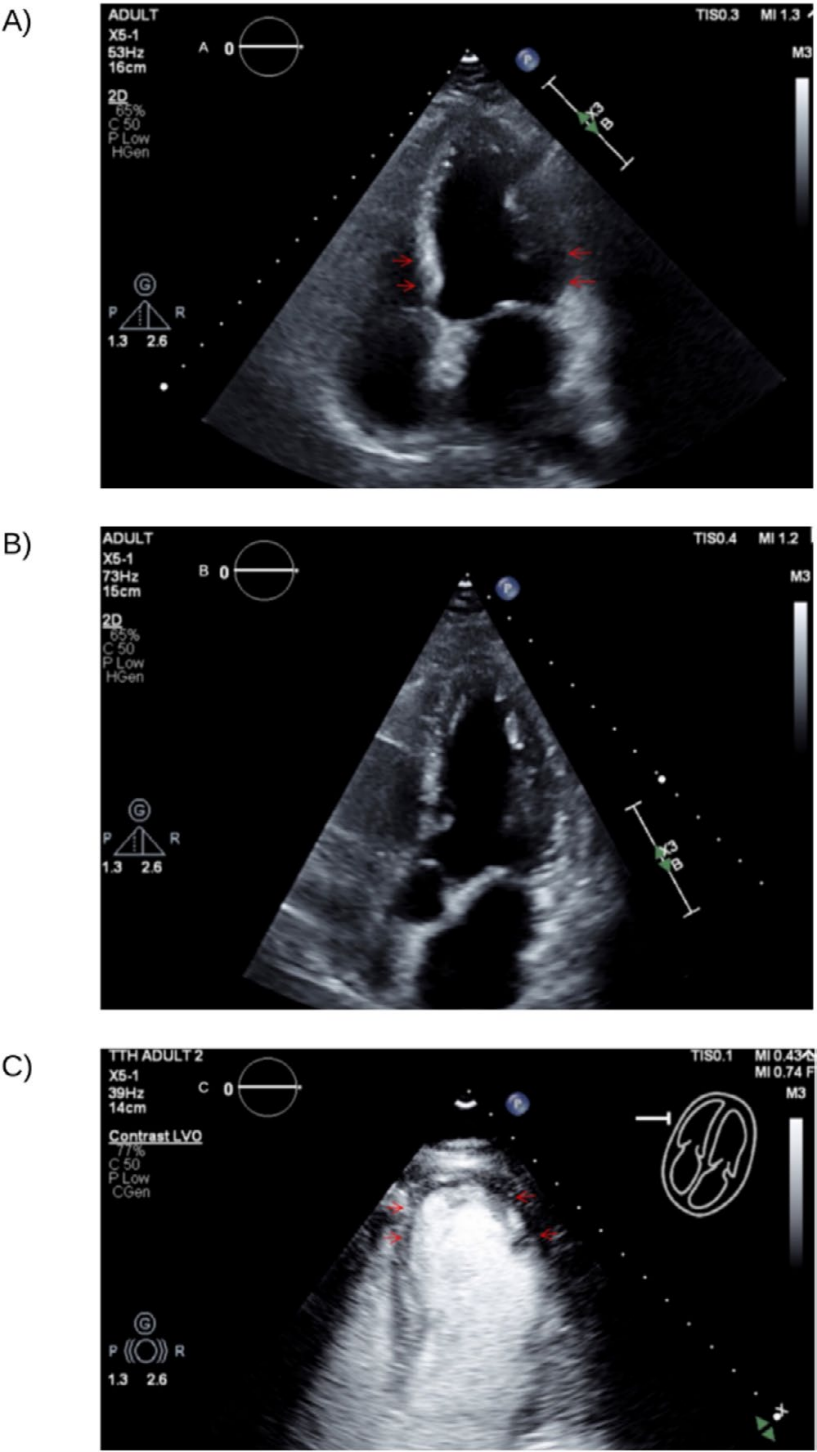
(A) Initial echocardiogram shows reverse takotsubo pattern with basal hypokinesis (red arrows) and apical sparing. (B) Subsequent echocardiogram shows the recovery of wall motion done after months. (C) Last echocardiogram demonstrates recurrence with typical takotsubo pattern of apical ballooning (red arrows) and basal hyperkinesis.
